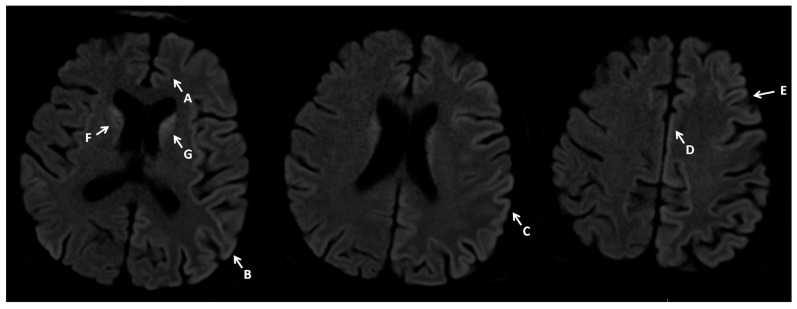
Contrast MRI imaging 7 February 2024. Imaging performed on a 1.5T scanner. Brain MRI shows increased signals on DWI diffusion images in the cerebral cortex—cortical ribbon sign (A–E) and caudate nuclei (F,G), with these changes being more pronounced in the left hemisphere.
